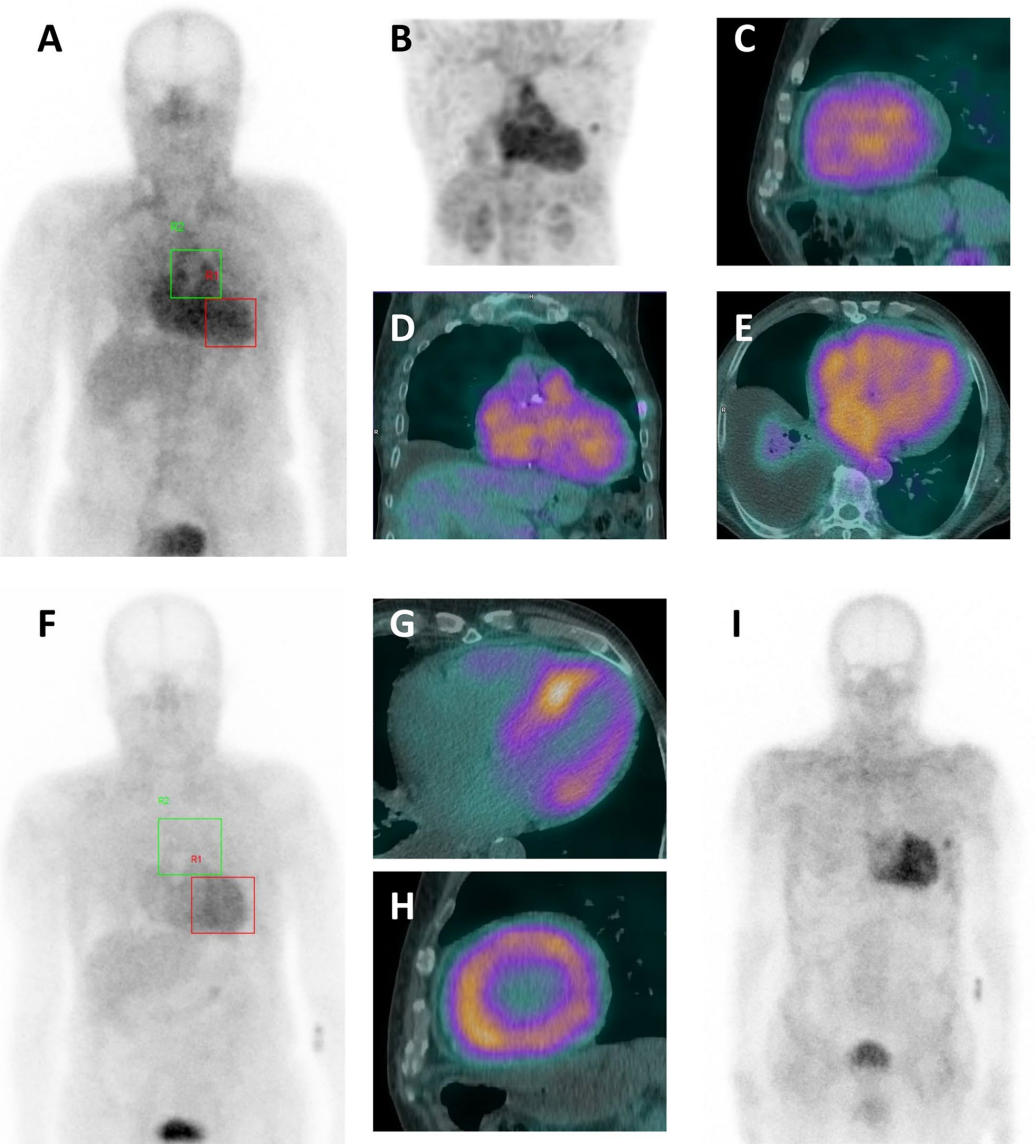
An example of true negative HMDP bone scintigraphy but positive DPD bone scintigraphy (patient #10). A, B Early ^99m^TC-HMDP-BS planar imaging (A) and a maximum intensity projection sequence (B) presenting diffuse uptake with an H/M ratio of 1.01. C–E Early ^99m^TC-HMDP-BS SPECT imaging and coupled CT, showing diffuse uptake by the blood pool but no cardiac uptake. F Early ^99m^TC-DPD-BS planar imaging with cardiac uptake and an H/M ratio of 1.56. G, H Early ^99m^TC-DPD-BS SPECT imaging and coupled CT, showing diffuse myocardial uptake. I Late ^99m^TC-DPD-BS planar imaging with cardiac uptake (Perugini score: 3)
